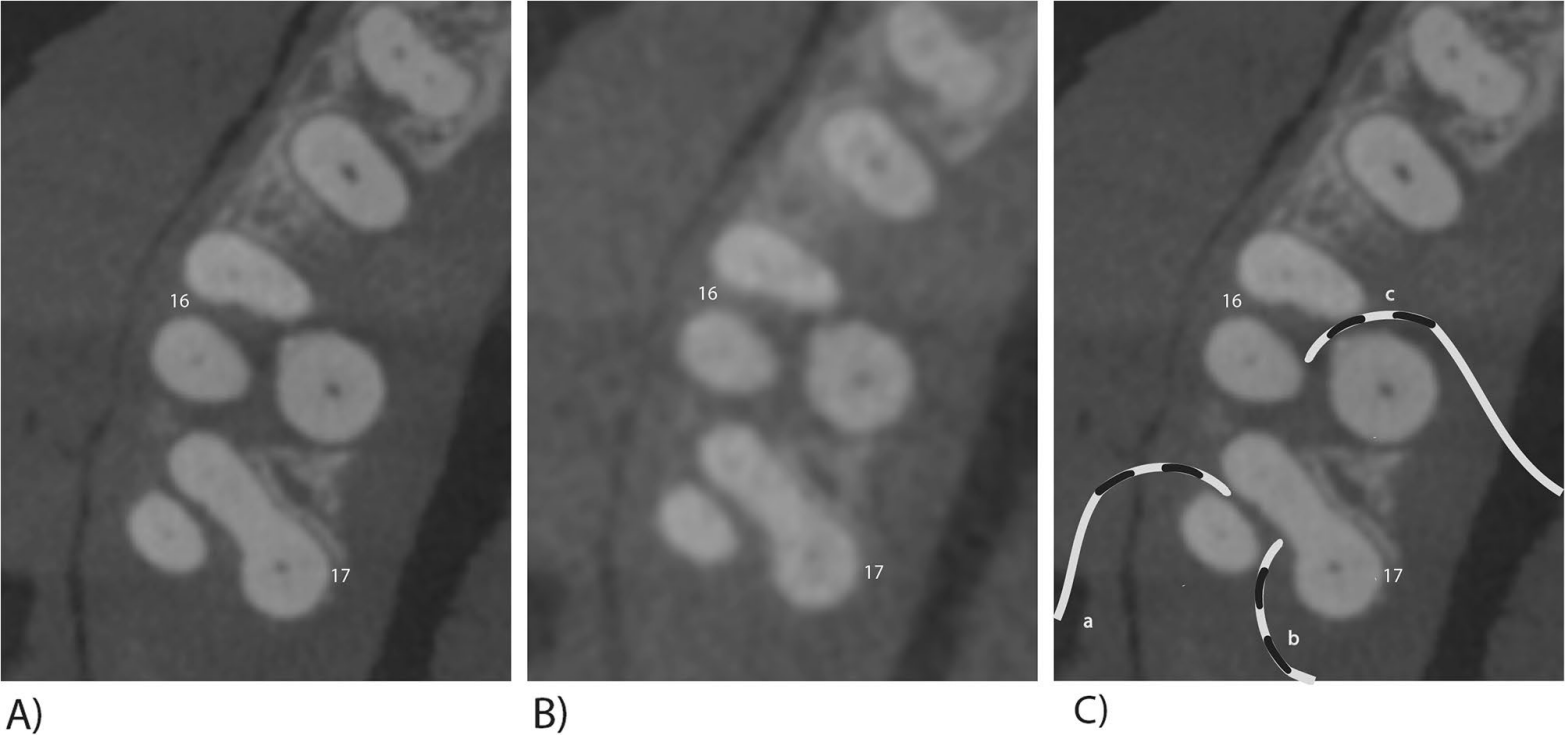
Different CBCT modes of teeth 16 and 17 with furcation defects. (A) HD-CBCT (B) LD-CBCT (C). a–c: different problematic intrasurgical probing angles leading to underestimation of the furcation defect.
